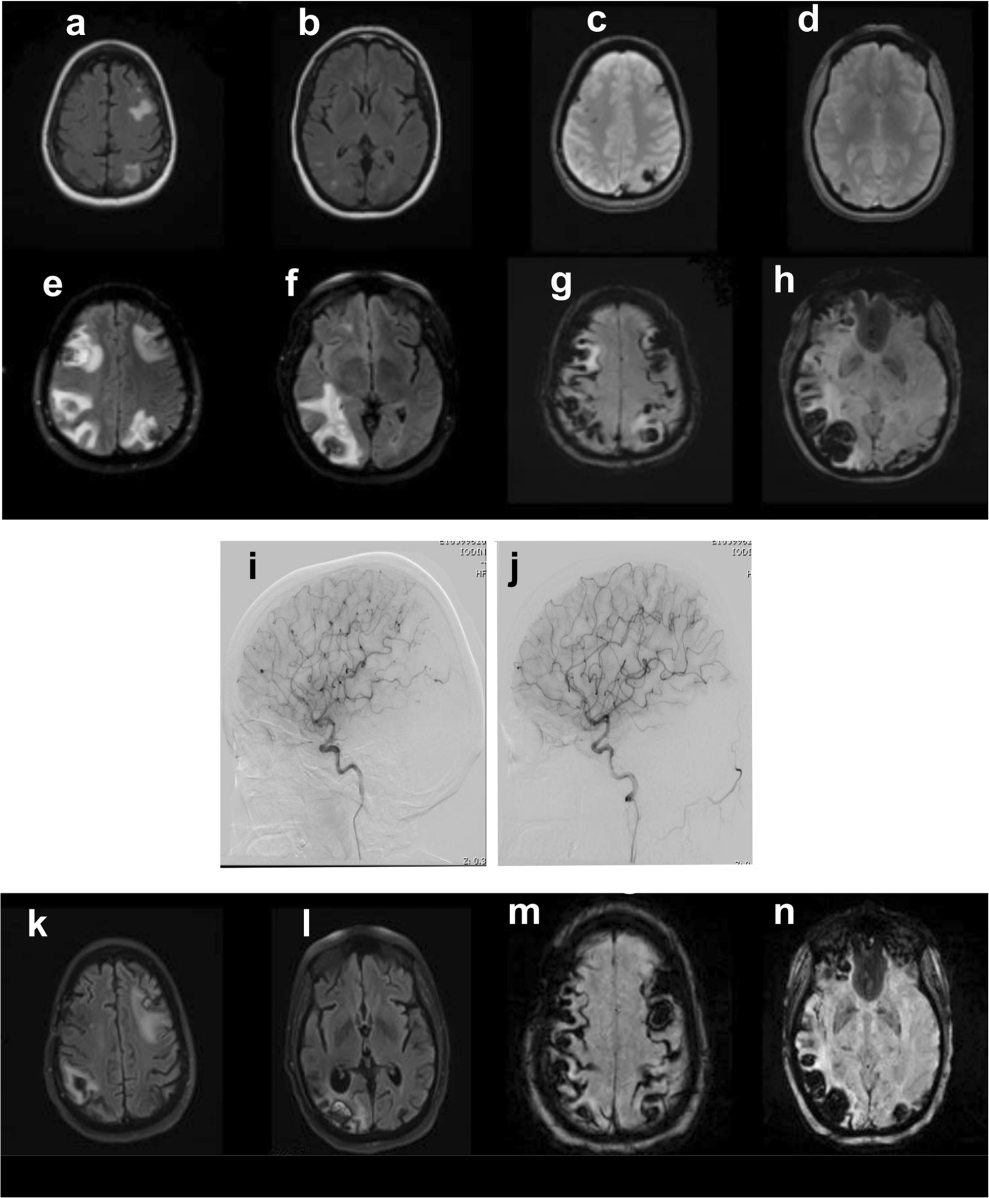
a–n Timeline of patient: representative images. Representative images from April 2017 (7 months after resection of atrial myxoma) with fluid-attenuated inversion recovery (a, b) and gradient recalled echo sequences (c, d) showing small hemorrhagic lesions throughout the bilateral frontal, parietal, and occipital lobes. Follow-up imaging from October 2017 shows interval increase in size of numerous lesions on fluid-attenuated inversion recovery (e, f) and susceptibility weighted imaging (g, h). i (right internal carotid artery) and j (left internal carotid artery) are representative images from cerebral angiogram done in October 2017, demonstrating multiple mycotic aneurysms. Fluid-attenuated inversion recovery (k, l) and susceptibility weighted imaging (m, n) sequences from repeat magnetic resonance imaging of the brain in March 2018 (post-radiation)
